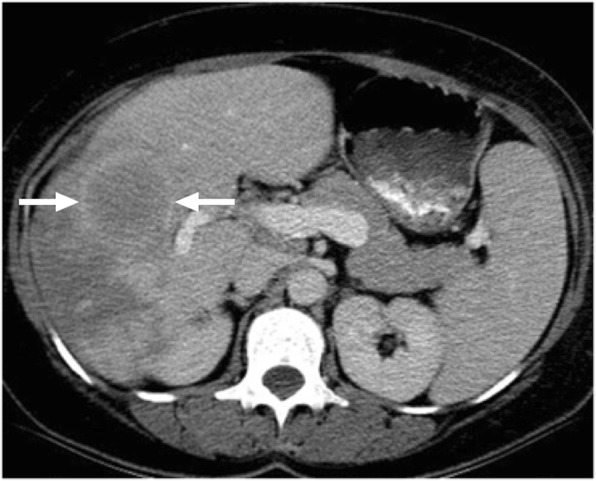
A 40-year-old female presenting with mild fever and liver mass on US examination. Axial plane postcontrast CT image demonstrates ill-defined, hypoattenuating mass (arrows) in the right lobe with accompanying wide parenchymal area of disturbed perfusion. Percutaneous biopsy revealed basophilic aggregates and sulfur granules consistent with actinomycetes infection. Post-antibiotic treatment demonstrated almost complete regression of the imaging findings (not shown)
